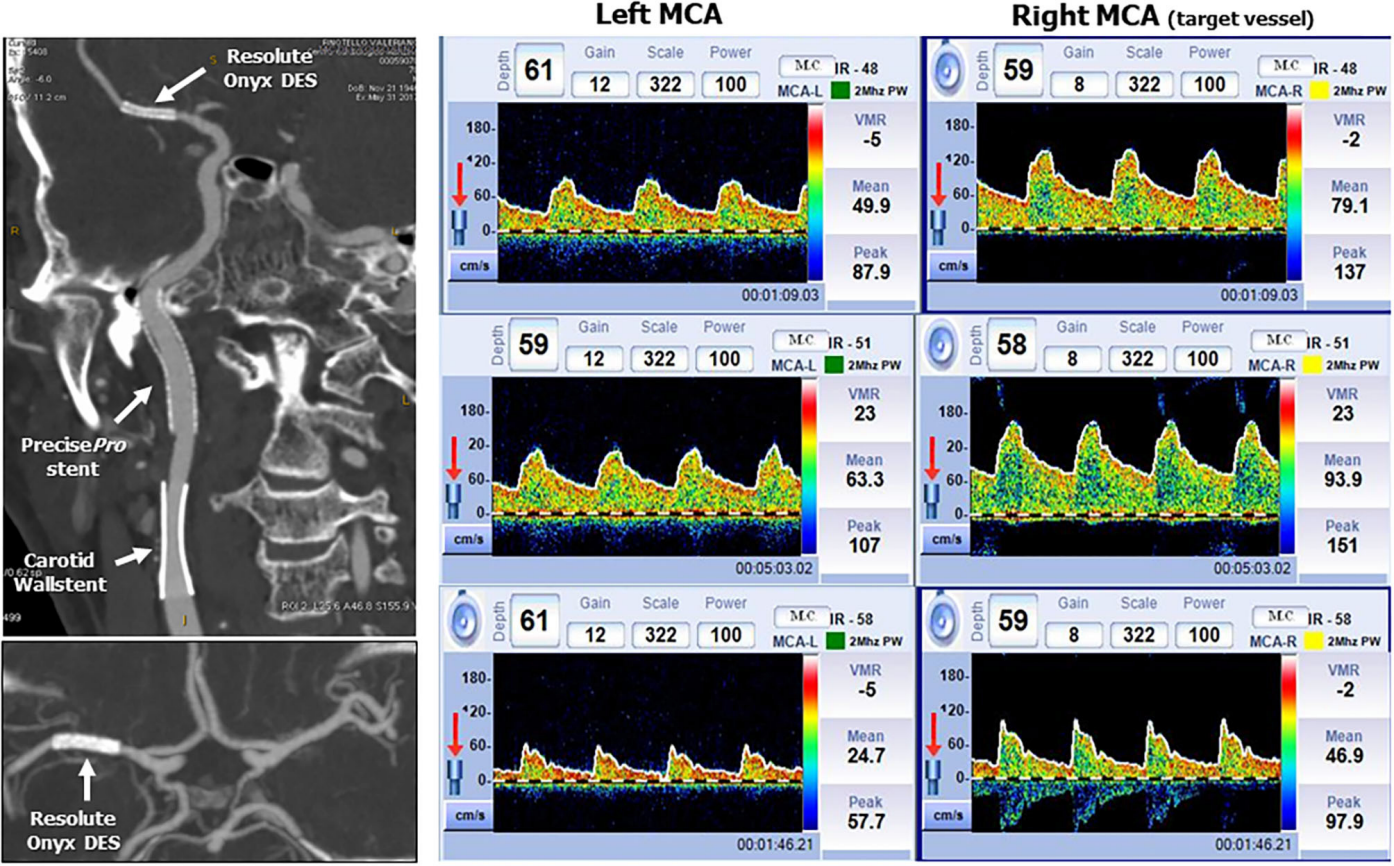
CT angiography and transcranial Doppler at 1-year follow-up. Left side: Patency of the three stents by multiplanar reconstruction with maximum intensity projection in the sagittal plane (upper image) and axial plane at the level of Willis circle (lower image). Right side: Upper strip: average peak systolic velocity in the left and right (target vessel) MCA. The right MCA PSV is 79 cm/sec indicating a <50% in-stent restenosis. Middle strip: Breath holding test. Left MCA breath holding index 0.82 and right breath holding index 0.75 (normal value >0.69). Lower strip: hyperventilation test: Left MCA average PSV reduction 51%. Right MCA average PSV reduction 40%.
